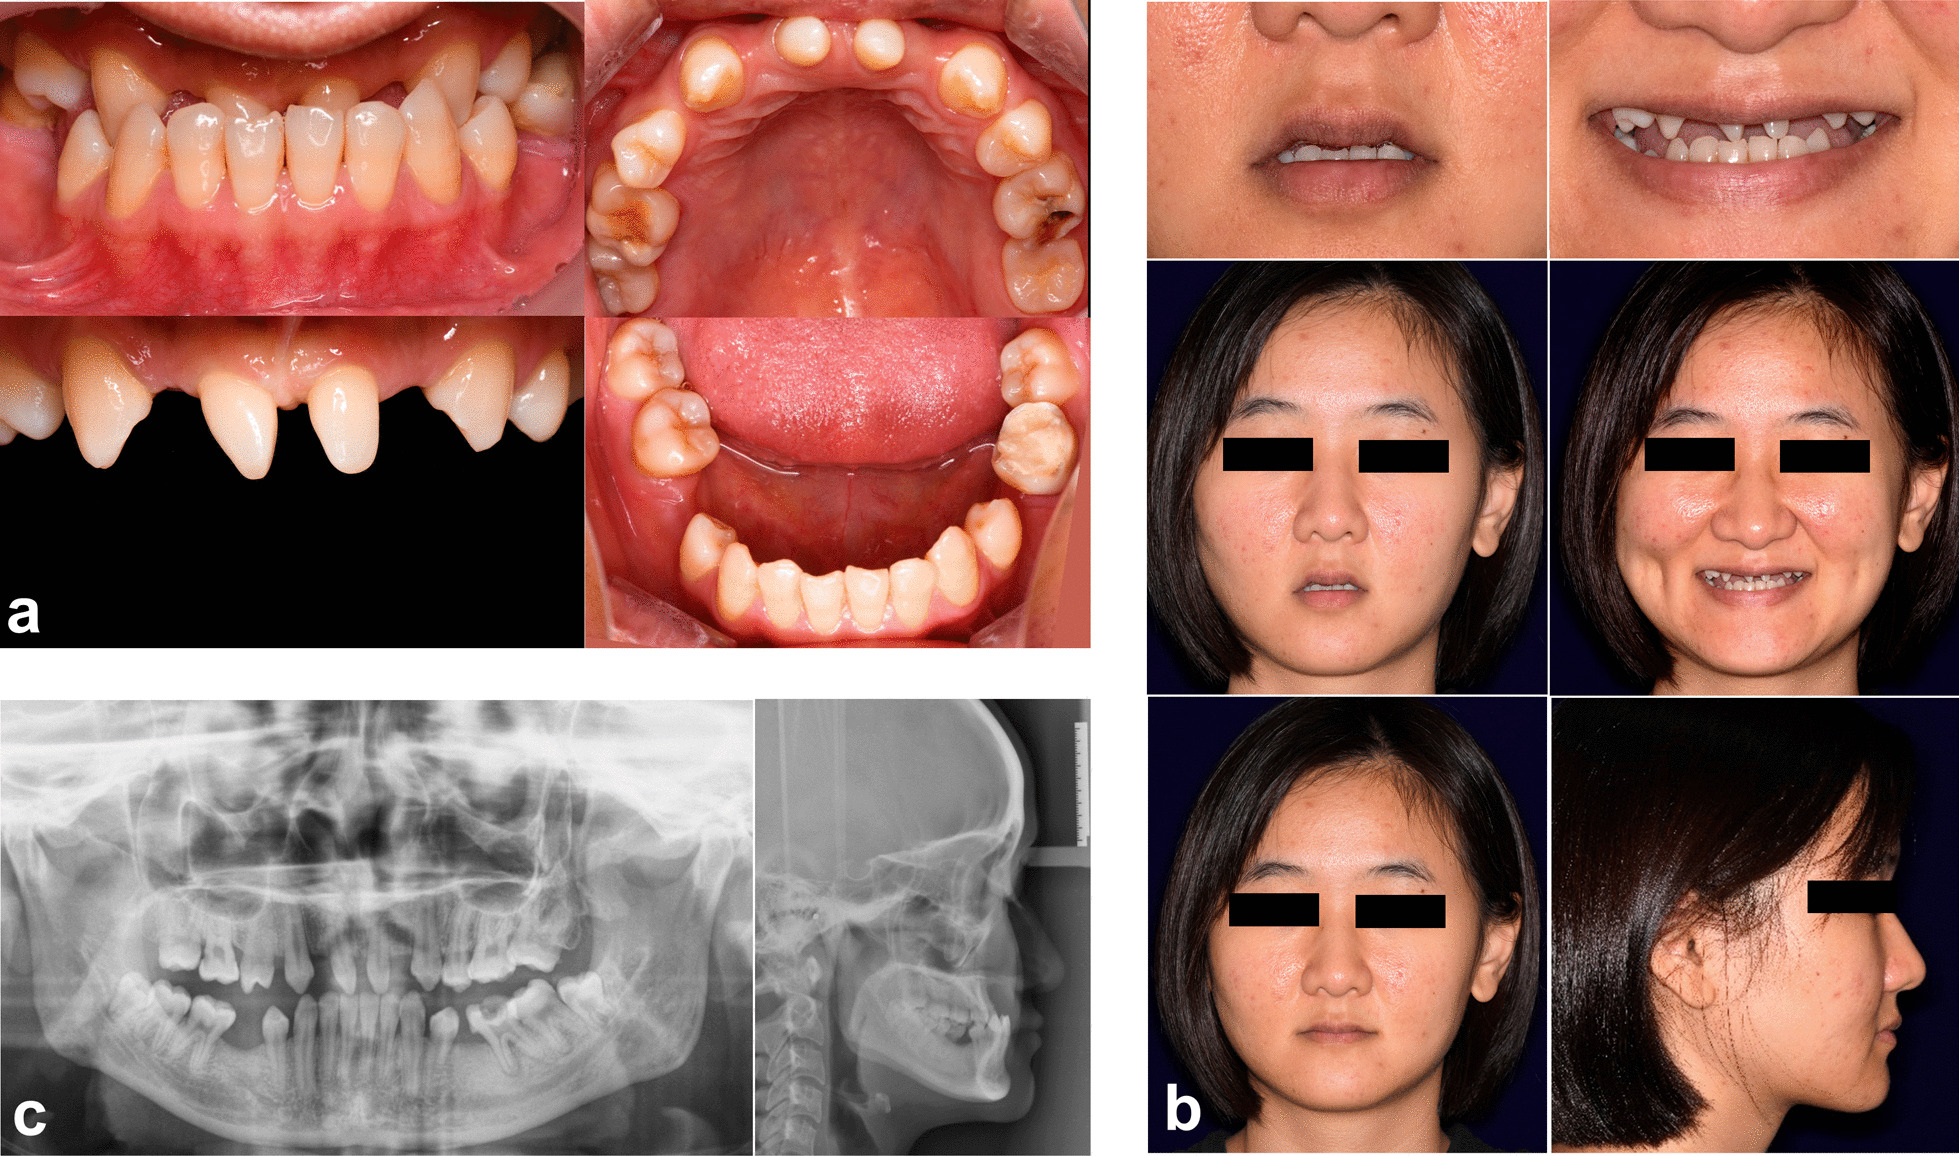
Initial intraoral, facial photographs and radiological examination. a Intraoral photographs; b facial photographs in rest position, wide smile, closed lips, and profile; c. panoramic radiography and cephalogram
